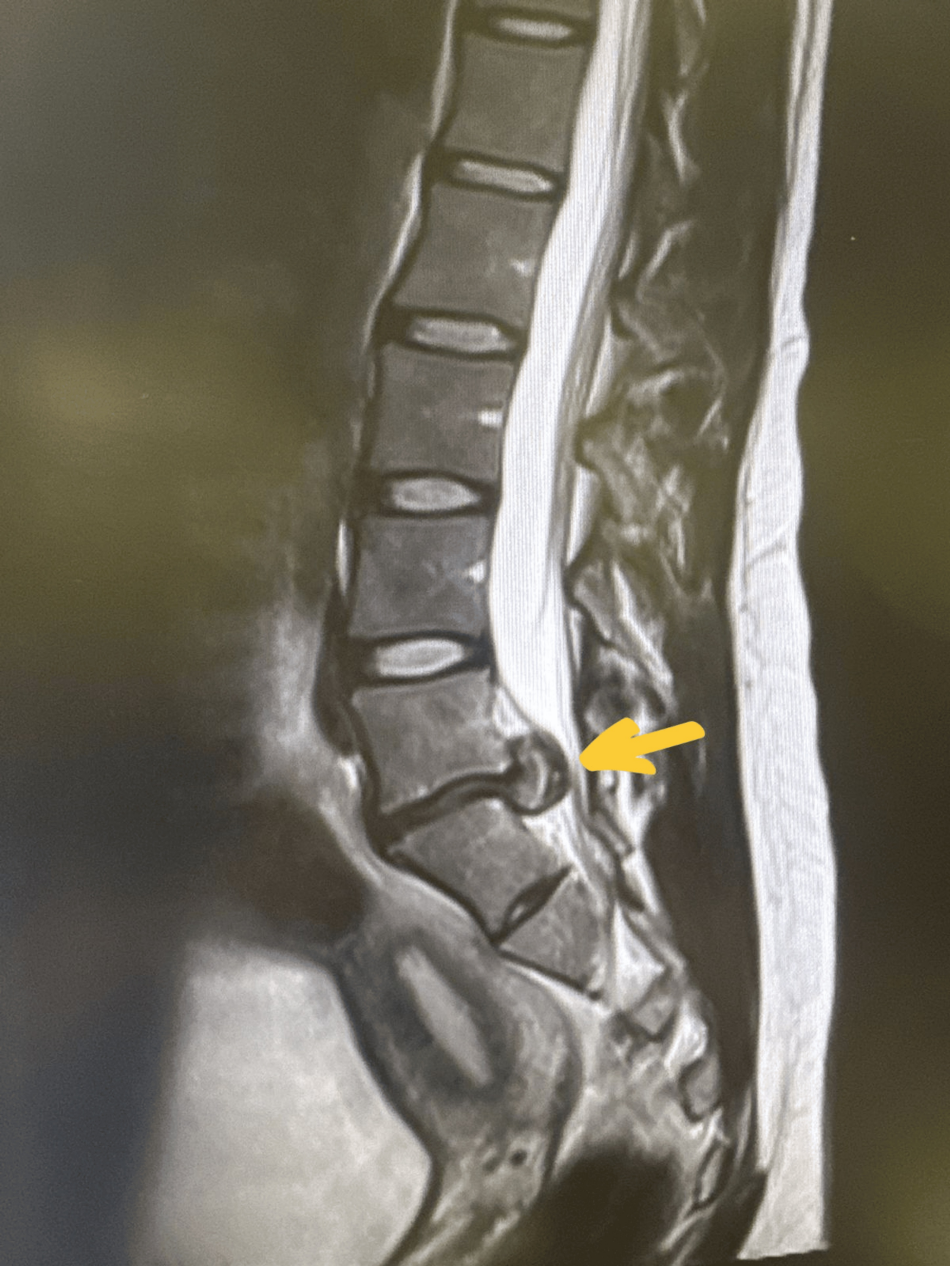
Sequential sagittal T2-weighted MRI image showing a massive central disc extrusion at L5–S1 with cranio-caudal migration (yellow arrow), causing severe compression of the cauda equina and bilateral S1 nerve roots.
